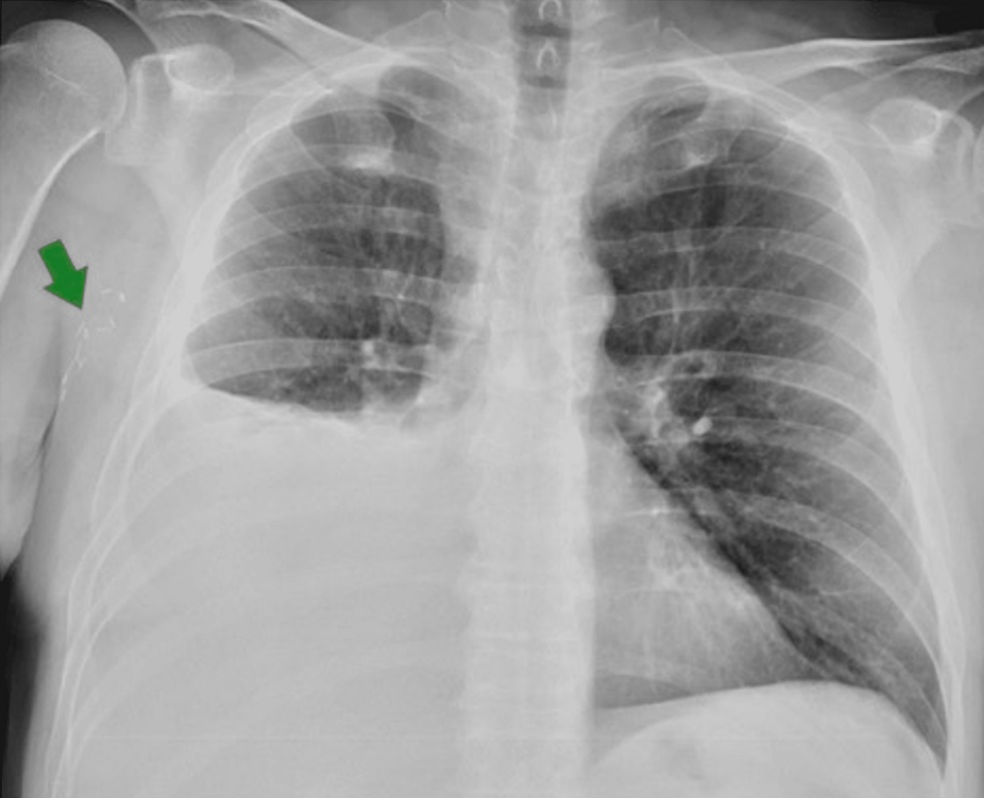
Anteroposterior chest X-ray on presentation. Large right-sided pleural effusion and surgical clips on the right chest wall after cutaneous melanoma resection (green arrow).
